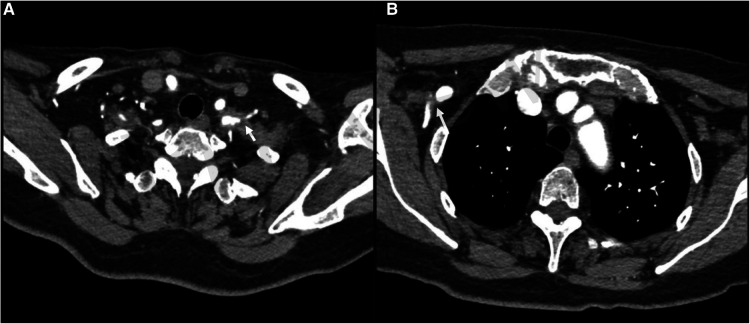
Preoperative CT scan revealing soft tissue compression of the right axillary artery (B) and the left subclavian and axillary artery (A).
